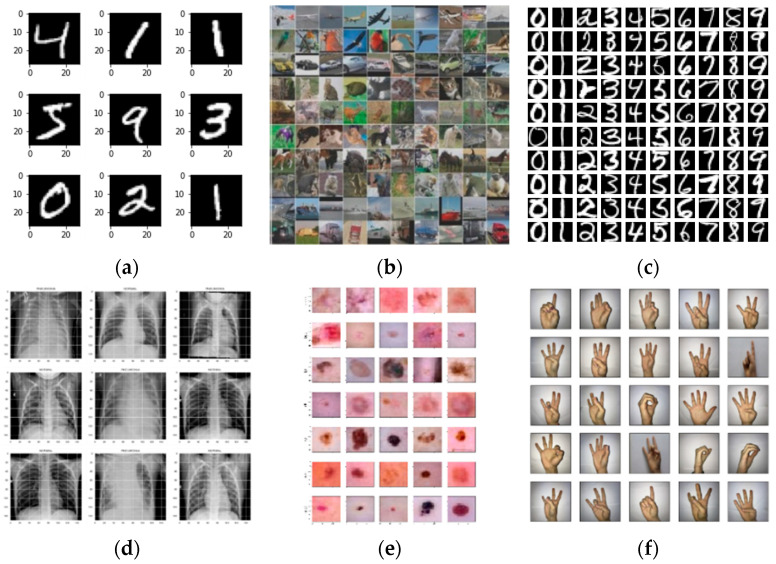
Sample images from: (a) MNIST, (b) STL-10, (c) USPS, (d) Pneumonia chest X-Ray, (e) DermaMNIST and (f) Digits-SL datasets.
